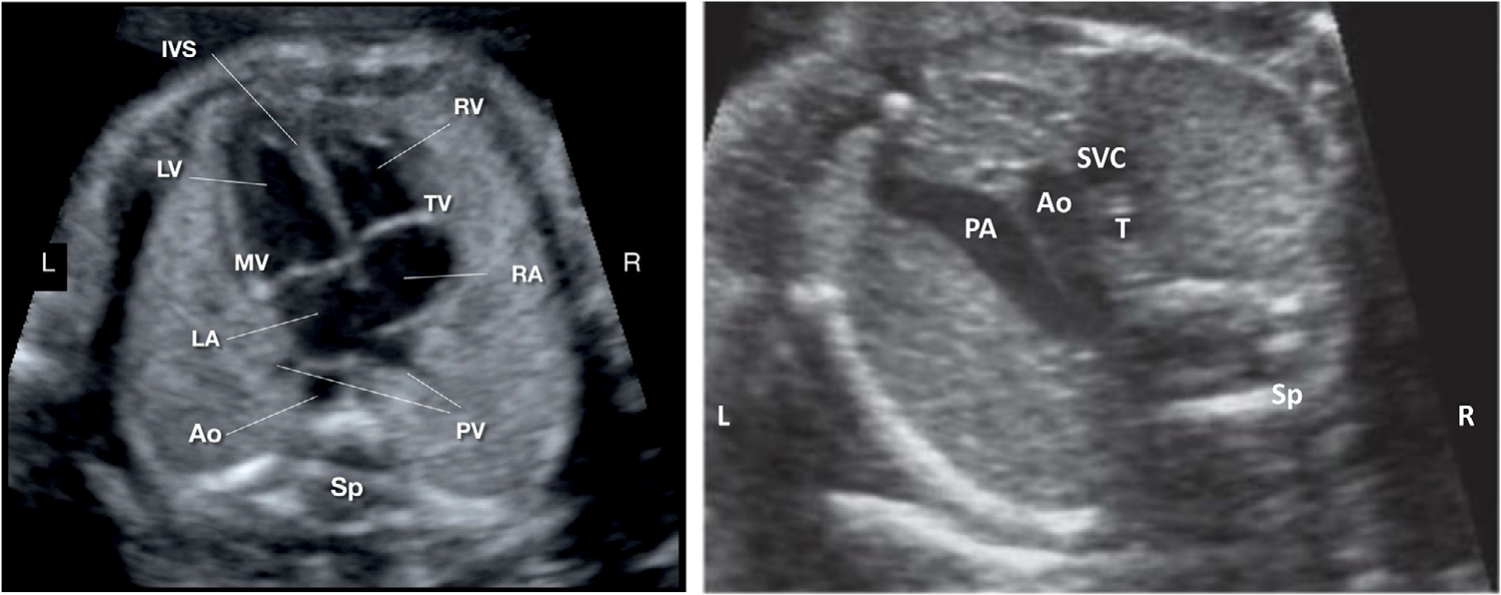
Ultrasound images of the fetal heart views used to screen for cardiac anomalies: the left‐hand image is of the 4‐chamber view and the right‐hand image is of the 3‐vessel and trachea view. Ao, aorta; IVS, interventricular septum; LA, left atrium; LV, left ventricle; MV, mitral valve; PV, pulmonary veins; RA, right atrium; RV, right ventricle; Sp, spine; SVC, superior vena cava; T, trachea; TV, tricuspid valve.
